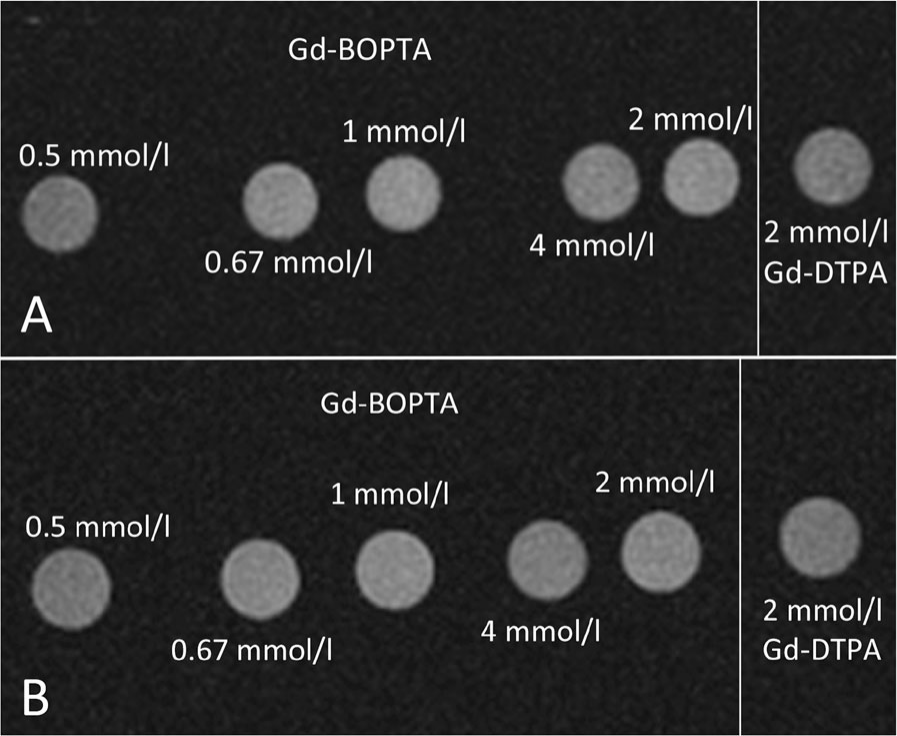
T1-weighted axial scan of two different sets of pipes containing different solutions of gadolinium-based contrast (a) with or (b) without addition of synovial fluid
